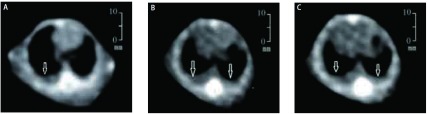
胸部CT连续观察同一只裸鼠胸腔积液形成。A：第7天见右侧胸腔积液；B：第14天出现双侧胸腔积液，体积较前增加；C：第21天见胸腔积液稍减少（箭头所示）。 Continuous observation of malignant pleural effusion via CT imagines on nude mise after LLC-EGFP cells inoculation. A: Malignant pleural effusion (MPE) was scaned in the right cavity at day 7 (arrow); B: A developing pleural effusion can be seen in the bilateral cavity at day 14 (arrows); C: The volume was slightly decreased at day 21 (arrows).
